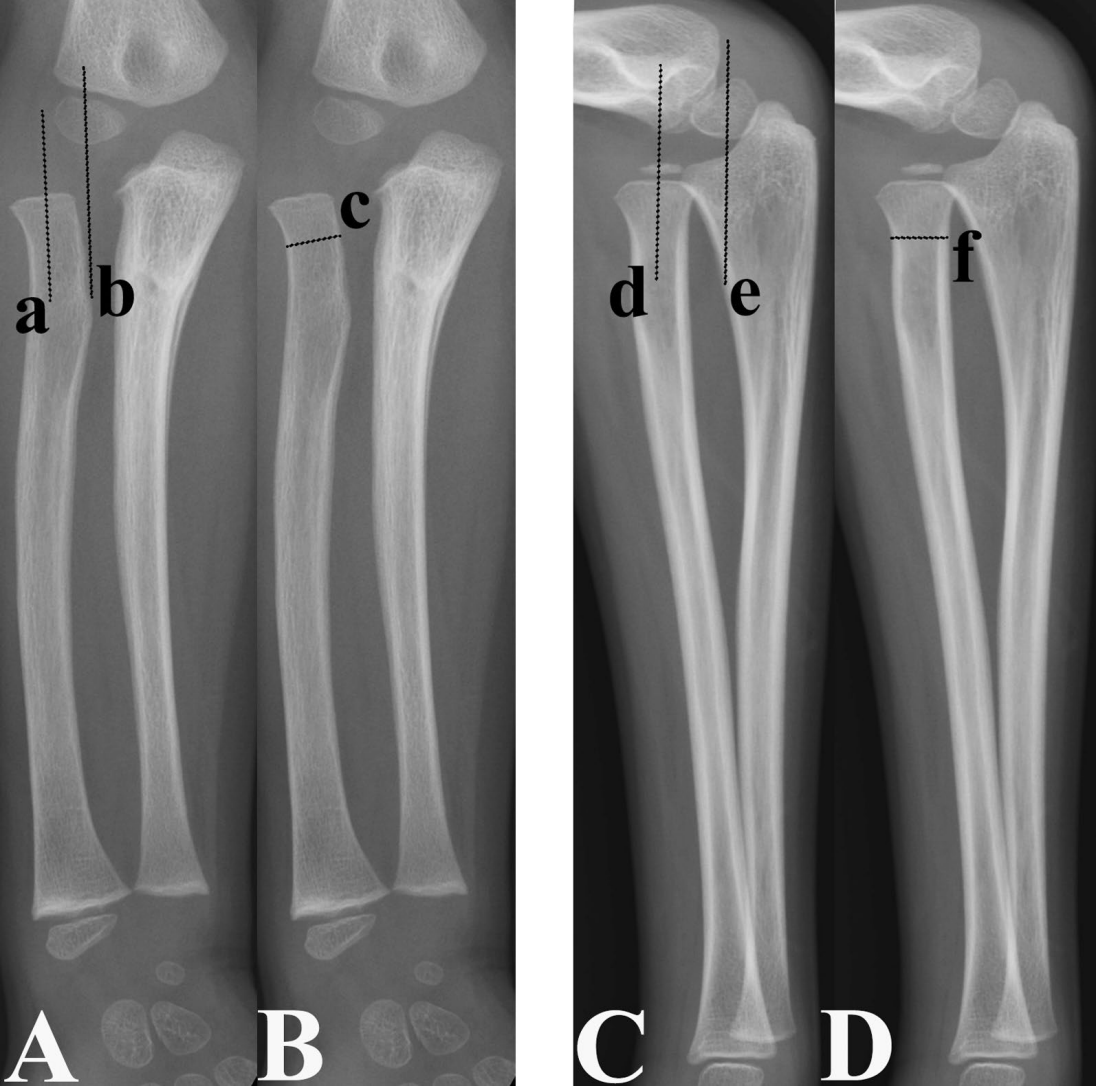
Measurement of the DD-RH on anteroposterior (A and B) and lateral (C and D) radiographs; DD-RH is expressed as the ratio of the distance between the forearm axes passing through the centre of the proximal radial metaphysis (a and d) and the centre of the humeral capitellum (b and e) to the narrowest radial neck width (lines c and f)
